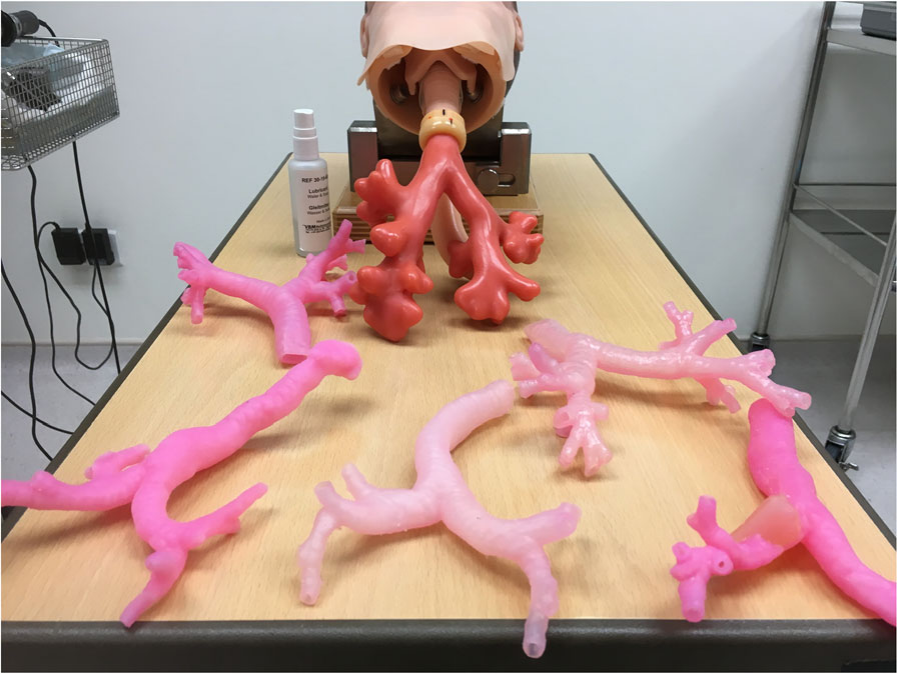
Standard airway trainer, comprising detachable lower airway model attached to upper airway structures. Surrounding this are a series of 3D printed models derived from CT scans as described in the Methods section
